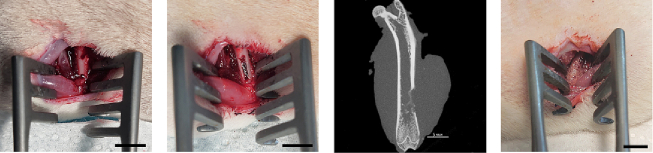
Representation of in vivo model. From left to right: surgical access to lateral surface of the femur bone and perforation of cavities; formation of longitudinal segmental defect in the compact bone and its inoculation with bacteria; X-ray visualization of the bone with the infected defect; treatment of the infected defect with bone cement material. Scale bar – 5 mm.
